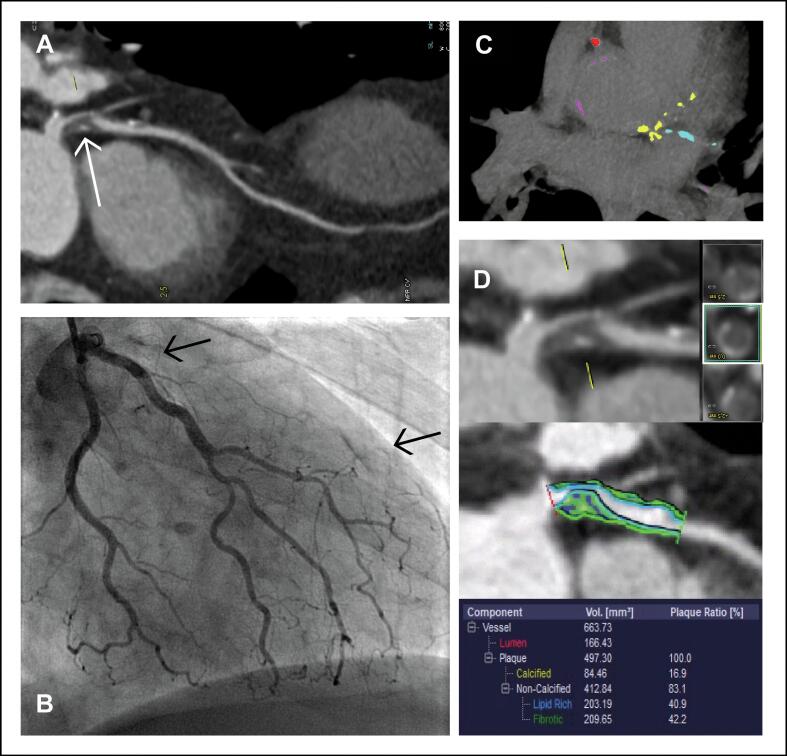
Patient example. Example of a patient presenting with non-ST-elevation myocardial infarction. A computed tomography angiography (panel A, C and D) was performed prior to invasive coronary angiography (panel B). Panel A: coronary artery stenosis (CAD-RADS 4A) of the proximal left anterior descending artery (white arrow). Panel B: proximal total occlusion of the left ascending descending artery with collateral filling (black arrows). Panel C: high Agatston score. Panel D: high-risk plaque with napkin-ring sign and low attenuation, and higher ratios of fibrosis and lipid compared to calcified (quantitative plaque analyses).
